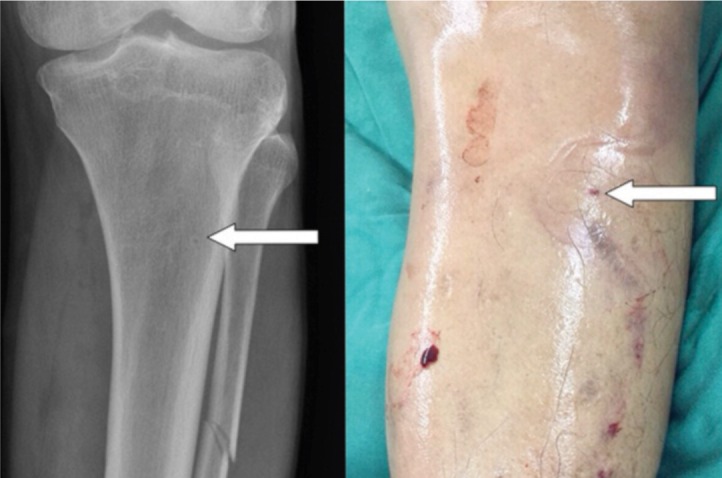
Radiograph and clinical photograph of swollen left leg prior to fasciotomy.
